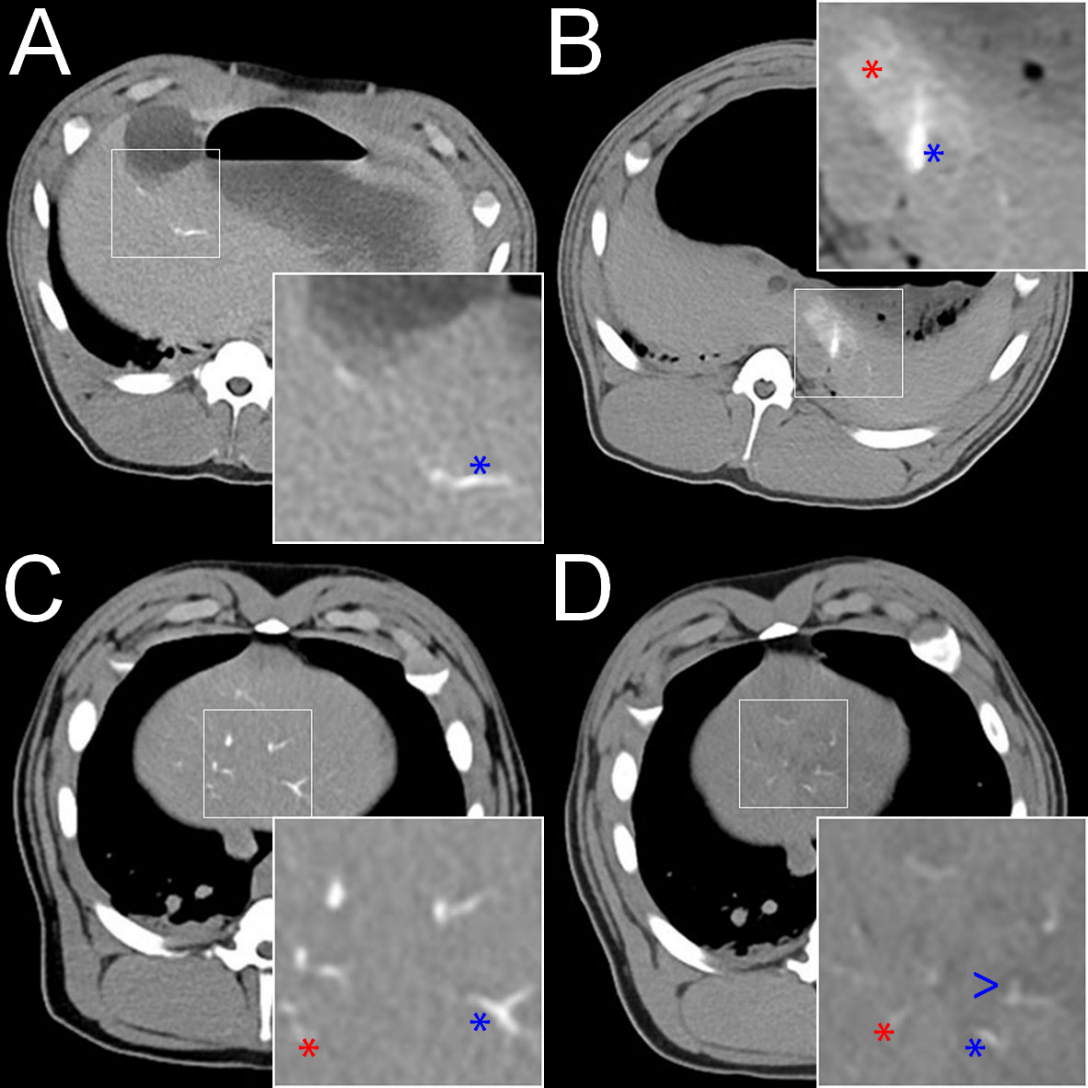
Radiopaque microspheres–standard CT findings. A RMS.a. Non-enhanced CT 2 hours after embolization. Marked linear hyperdensity (blue asterisk) corresponding to an occluded subsegmental artery (arterial enhancement). The type of arterial enhancement was comparable to iodized oil. B RMS.sa. Non-enhanced CT 2 hours after embolization. Linear (blue asterisk) and patchy hyperdensities (red asterisk) corresponding to occluded subsegmental and interlobular arteries and sinusoids (arterial and parenchymal enhancement). Note the more intense arterial and parenchymal enhancement compared to A. The type of arterial and parenchymal enhancement was comparable with iodized oil. C RMS-HEP.sa. Non-enhanced CT 2 hours after embolization. Note the more intense arterial (blue asterisk) and parenchymal enhancement (red asterisk) than A and B. D RMS-HEP.sa. Non-enhanced CT 7 days after embolization. Note the weaker arterial (blue asterisk) and parenchymal (red asterisk) enhancement compared to C. Note the hypodense areas in the embolized liver parenchyma (blue arrowhead) representing tissue necrosis.
